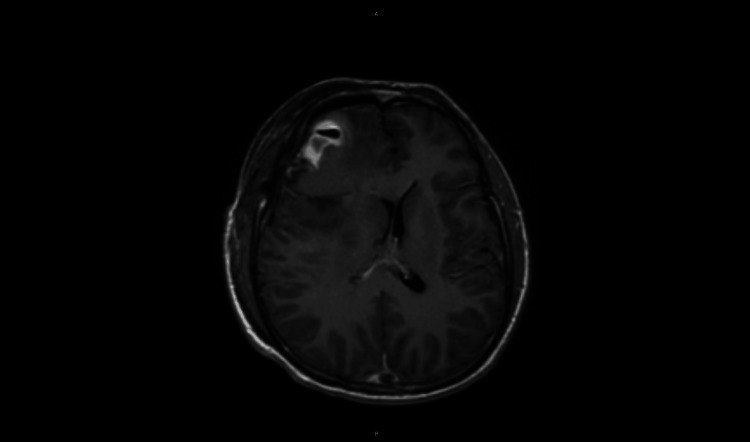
Postoperative MRI showing residual tumor and ischemic changes.
